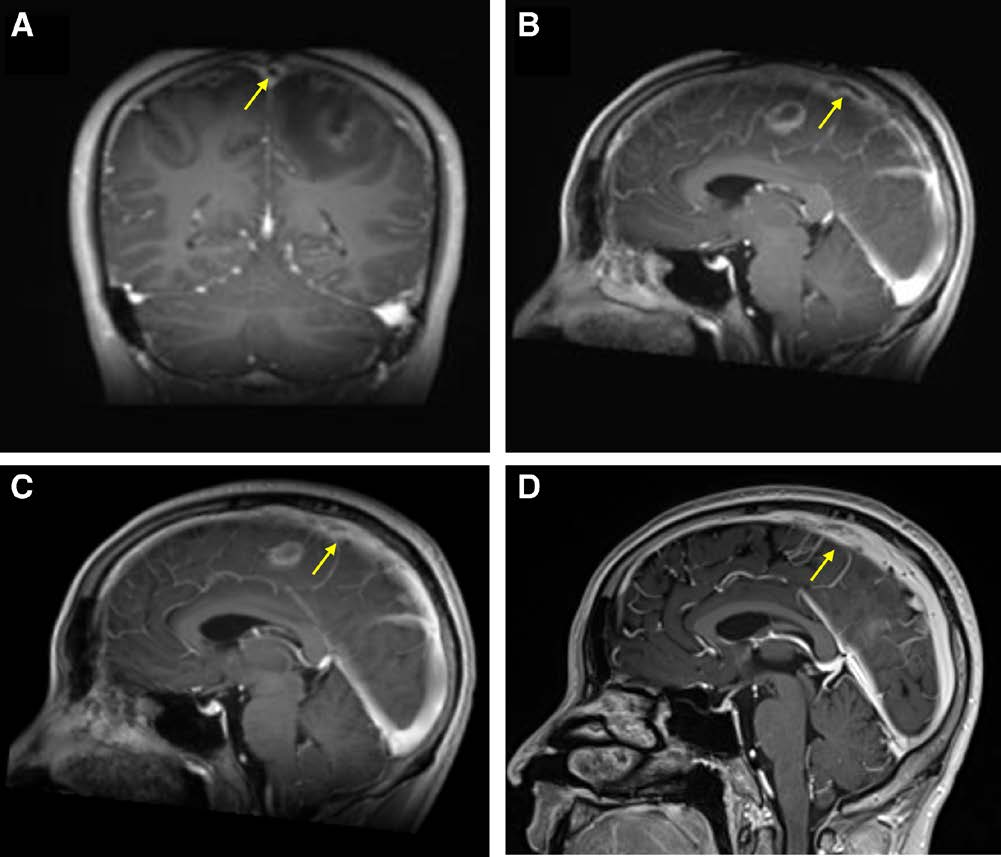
Images before and after the treatment. (A) Enhanced MRI showed irregular filling defect of middle superior sagittal sinus on T1WI coronary view before the treatment. (B) Enhanced MRI showed irregular filling defect of middle superior sagittal sinus on T1WI sagittal view before the treatment. (C) Enhanced MRI showed irregular filling defect of middle superior sagittal sinus on T1WI coronary view after the treatment. (D) Enhanced MRI showed irregular filling defect of middle superior sagittal sinus on T1WI sagittal view after the treatment. MRI = magnetic resonance imaging, T1WI = T1-weighted imaging.
